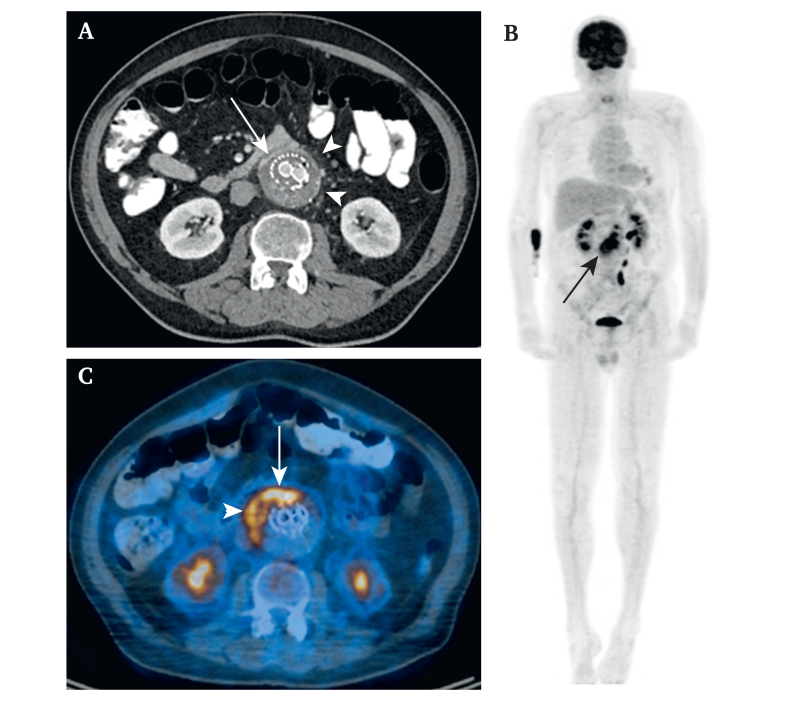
Multislice computed tomography angiography. (A) Transverse plane: peri-aortic enhancement (arrow), peri-aortic fat stranding (arrow heads). (B) Maximum intensity projection and (C) fused axial positron emission tomography CT images: intense, non-homogeneous fludeoxyglucose uptake of the stent graft (arrow in B and C) and of the thrombotic material in the aneurysm sac (arrow in B, arrowhead in C). Credits: Department of Nuclear Medicine and Radiology, Lucerne Cantonal Hospital.
